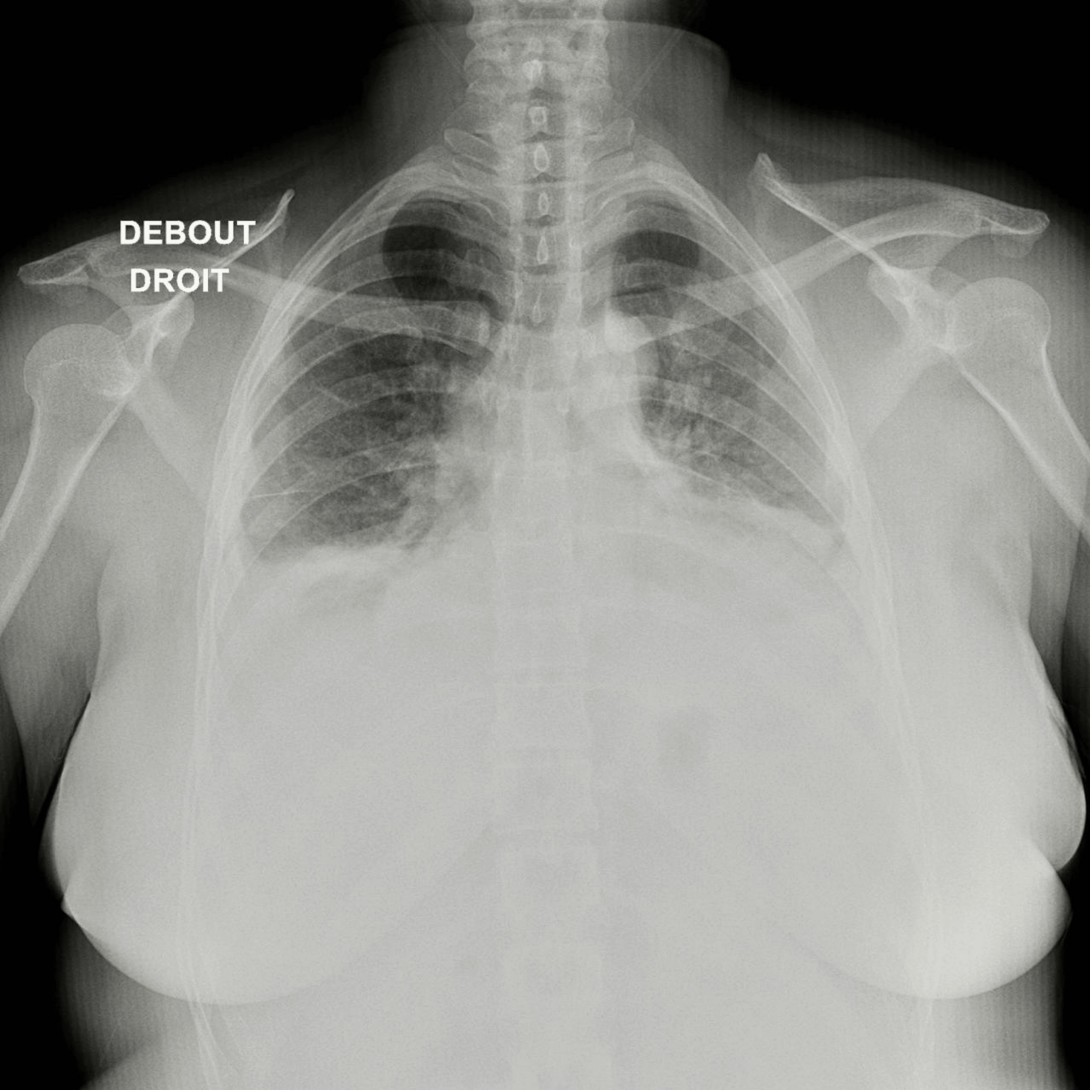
The chest X-ray showed an enlarged heart size with a classical batwing peri-hilum pattern sign and a pleural effusion.
